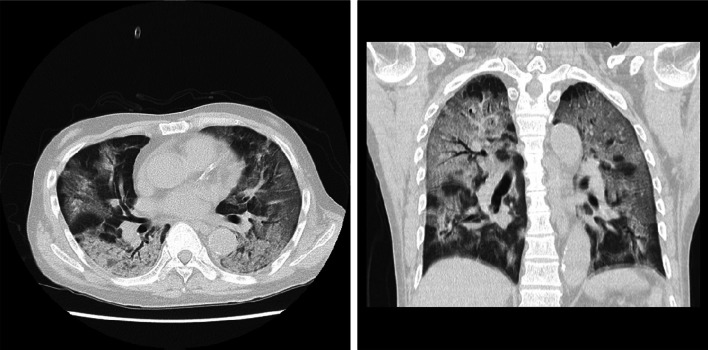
Chest computed tomography scan on admission
